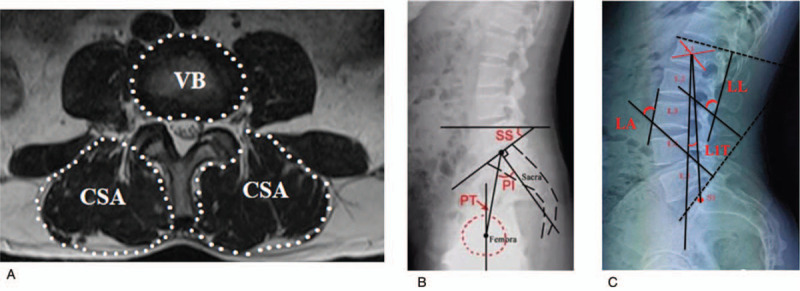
In X-ray and MRI Image, measurements of sagittal parameters of spinopelvic alignment (A and B) and the cross sectional-area (CSA) of the erector spinae (combined liocostalis and longissimus) and the vertebral body size (VB) on the inferior endplate of L1,2,3,4,5 axial (C). LL = lumbar lordosis, LA = lumbar vertebra angle, T1T = Lumbar 1 vertebra tilt angle. PI = pelvic incidence, PT = pelvic tilt, SS = sacral slope.
